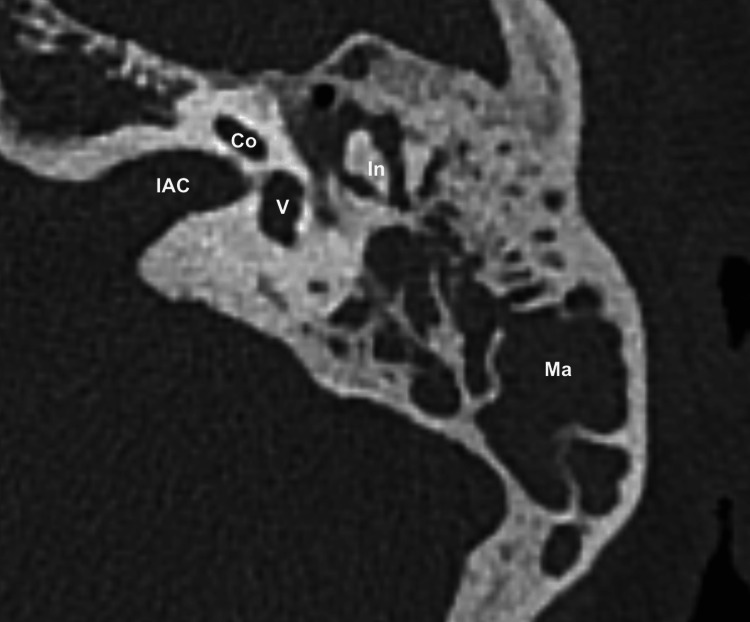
High-resolution CT of the left temporal bone showing an obstructed mastoid cavity and an obstructed tympanic cavity with no signs of erosion of the ossicles Co: Cochlea, V: Vestibule, In: Incus, IAC: Internal auditory canal, Ma: Mastoid
